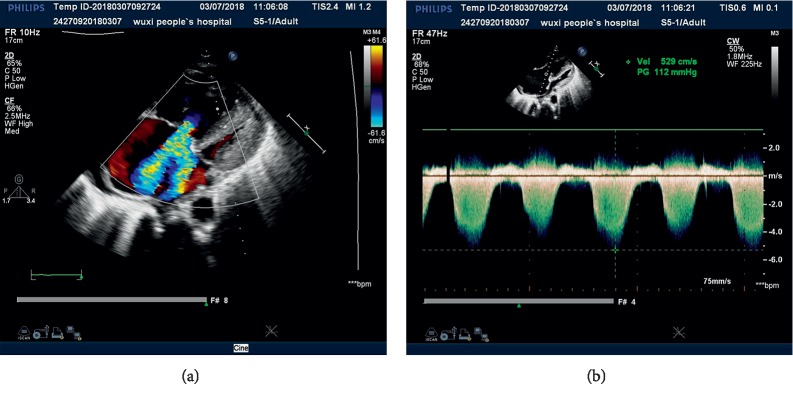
(a) Transthoracic echocardiography in patient 2 depicted obvious widening of the right atrium and right ventricle and severe regurgitation of the tricuspid valve. The pulmonary artery was distinctly broadened. (b) Pulmonary artery systolic pressure increased to up to 118 mmHg.
